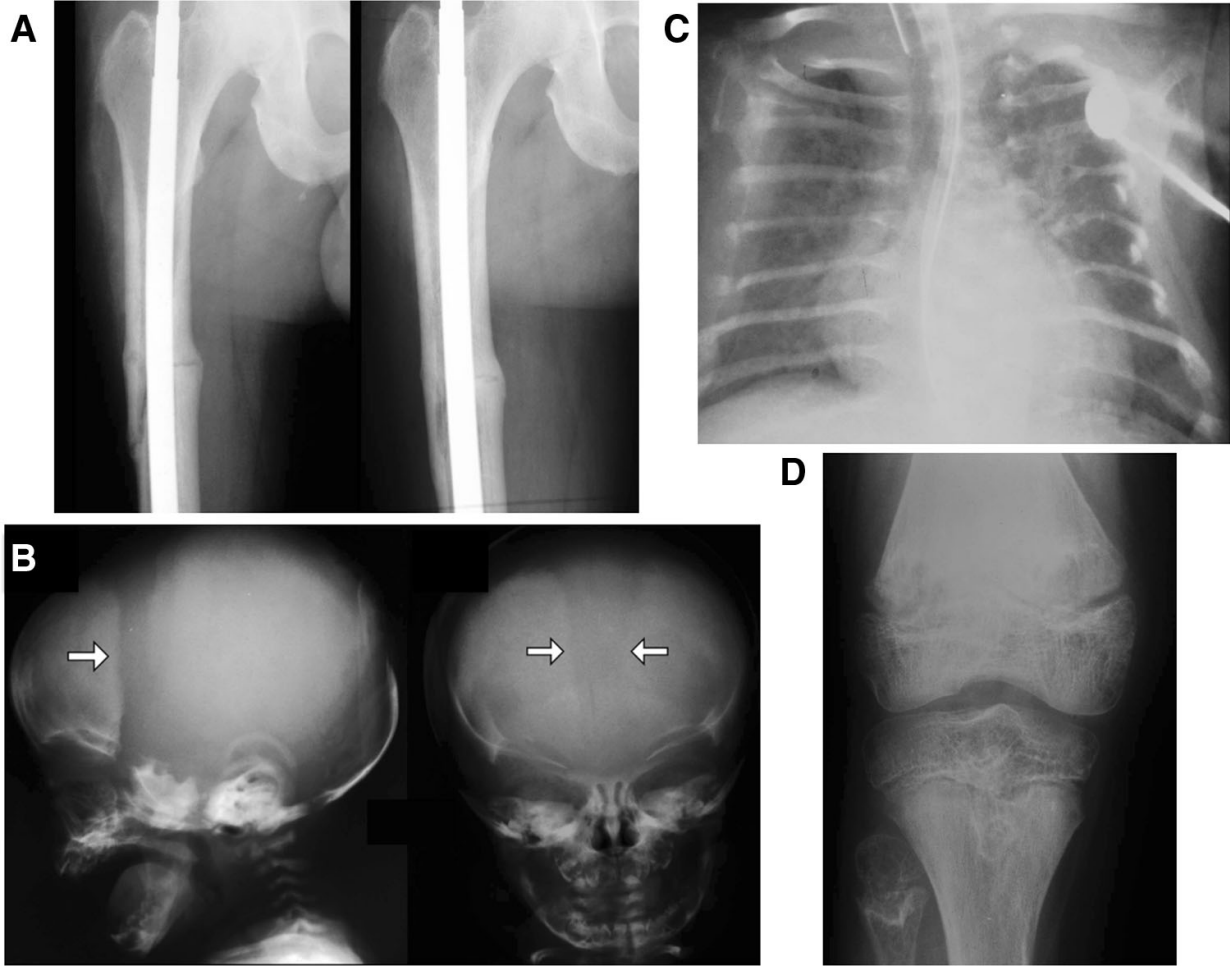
Radiographic images of HPP patients. a This middle-aged man who manifested signs and symptoms of hypophosphatasia during childhood shows some healing of a right femoral pseudofracture treated with intramedullary fixation. b Lateral and anteroposterior radiograph of the skull of this 14-week-old baby with infantile hypophosphatasia shows characteristic hypomineralization of areas of the calvarium that give the appearance of widened sutures (*arrows*). c At 23 weeks-of-age, this radiograph of the chest of a baby with infantile hypophosphatasia shows gracile, deformed, and fractured ribs that contribute to the respiratory compromise that is often lethal for such patients. d This radiograph of the right knee of a 9-year-old girl with childhood hypophosphatasia shows characteristic findings including areas of osteopenia and osteosclerosis especially in the metaphyseal regions, and marked physeal widening and irregularity of the head of the fibula
